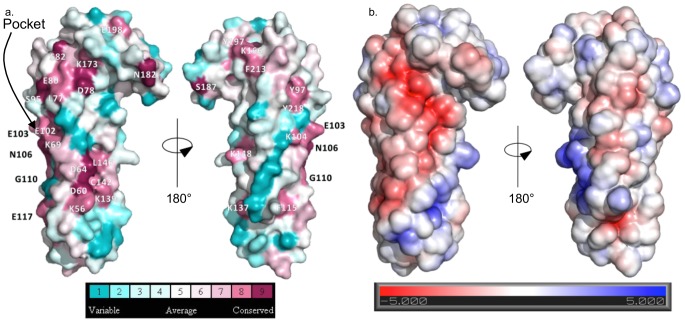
Surface of *Pb*SPECT1Δ41. a. Residues conserved among homologs of *Pb*SPECT1 were mapped to the molecular surface of *Pb*SPECT1Δ41. Two views of *Pb*SPECT1 related by a 180° rotation around the indicated axis are shown. Color scale for conservation is shown at bottom. b. The electrostatic surface mapped to the van der Waals surface of *Pb*SPECT1Δ41 is shown. As in panel a, two views and a color scale are shown.
